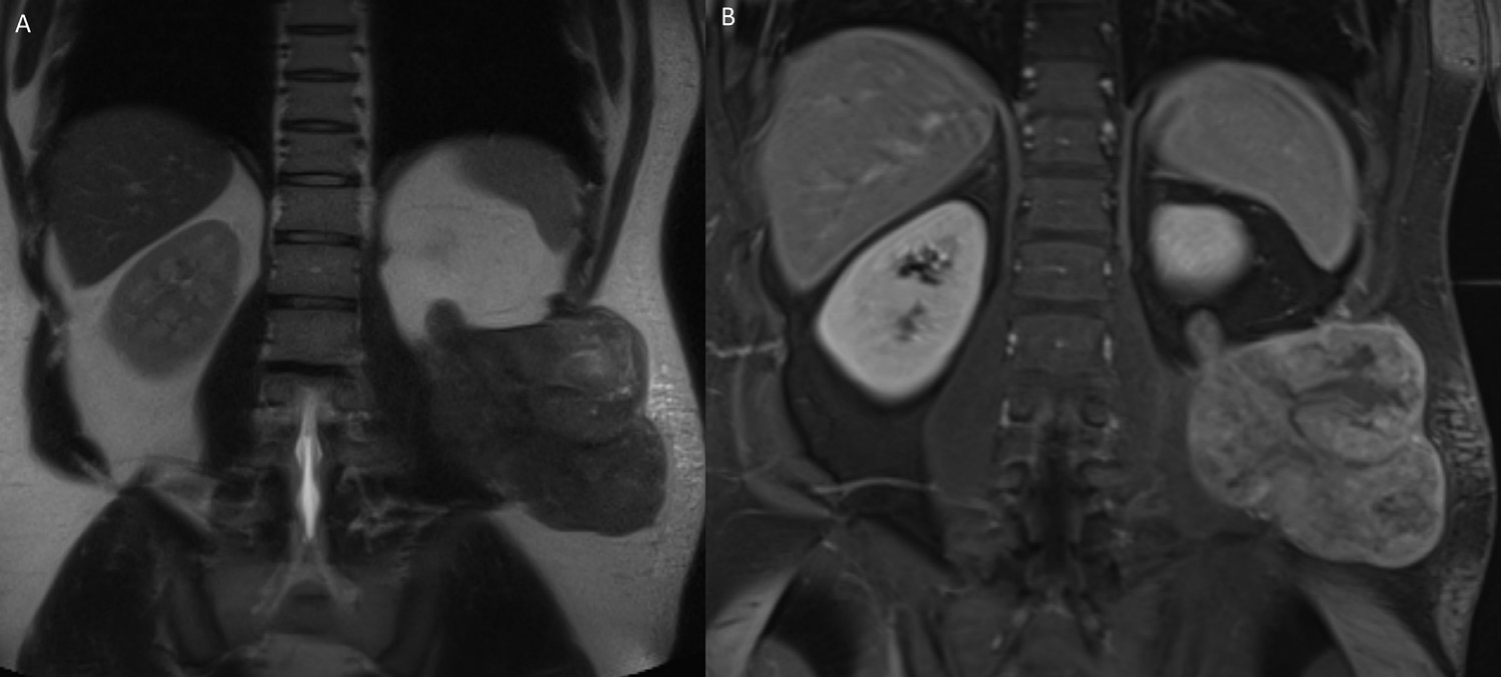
Two years following resolution of left iliopsoas mass, magnetic resonance imaging (MRI) of the abdomen, including T2 Half-Fourier Acquisition Single-Shot Turbo Spin Echo (HASTE) Coronal (Fig. 5A) and Postcontrast T1 Volumetric Interpolated Breath-hold Examination (VIBE) Coronal (Fig. 5B) images, was performed for diagnostic workup of new onset of left flank pain. This study showed a large, partially enhancing mass in the left retroperitoneal space, invading the left iliopsoas muscle and extends into the left flank soft tissues, reflecting recurrence of metastatic disease.
